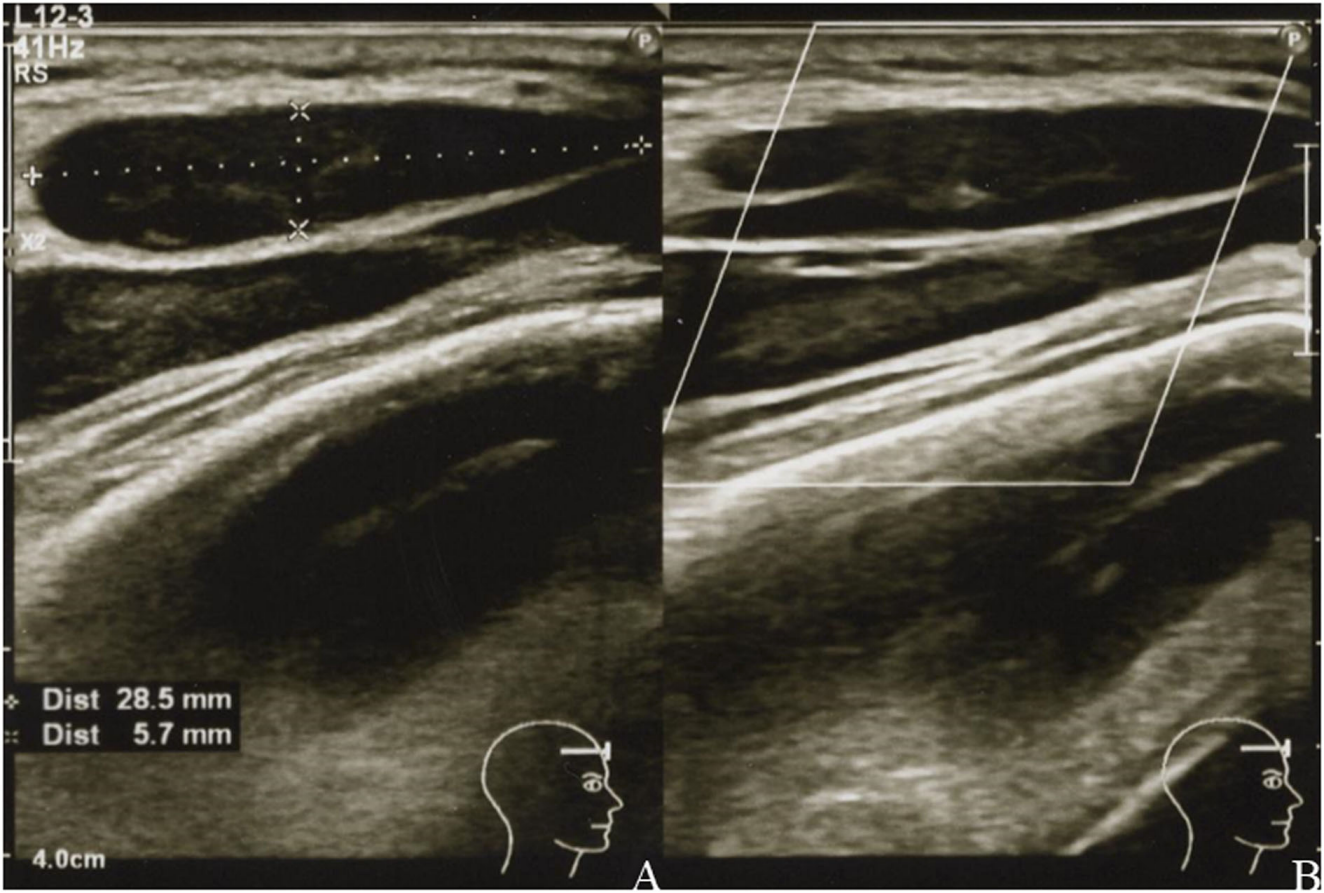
Grayscale (A) and color Doppler (B) images of the right temporal mass showing a uniformly hypoechoic teardrop-shaped mass with well-defined margins (A) and decreased vascularity (B).
